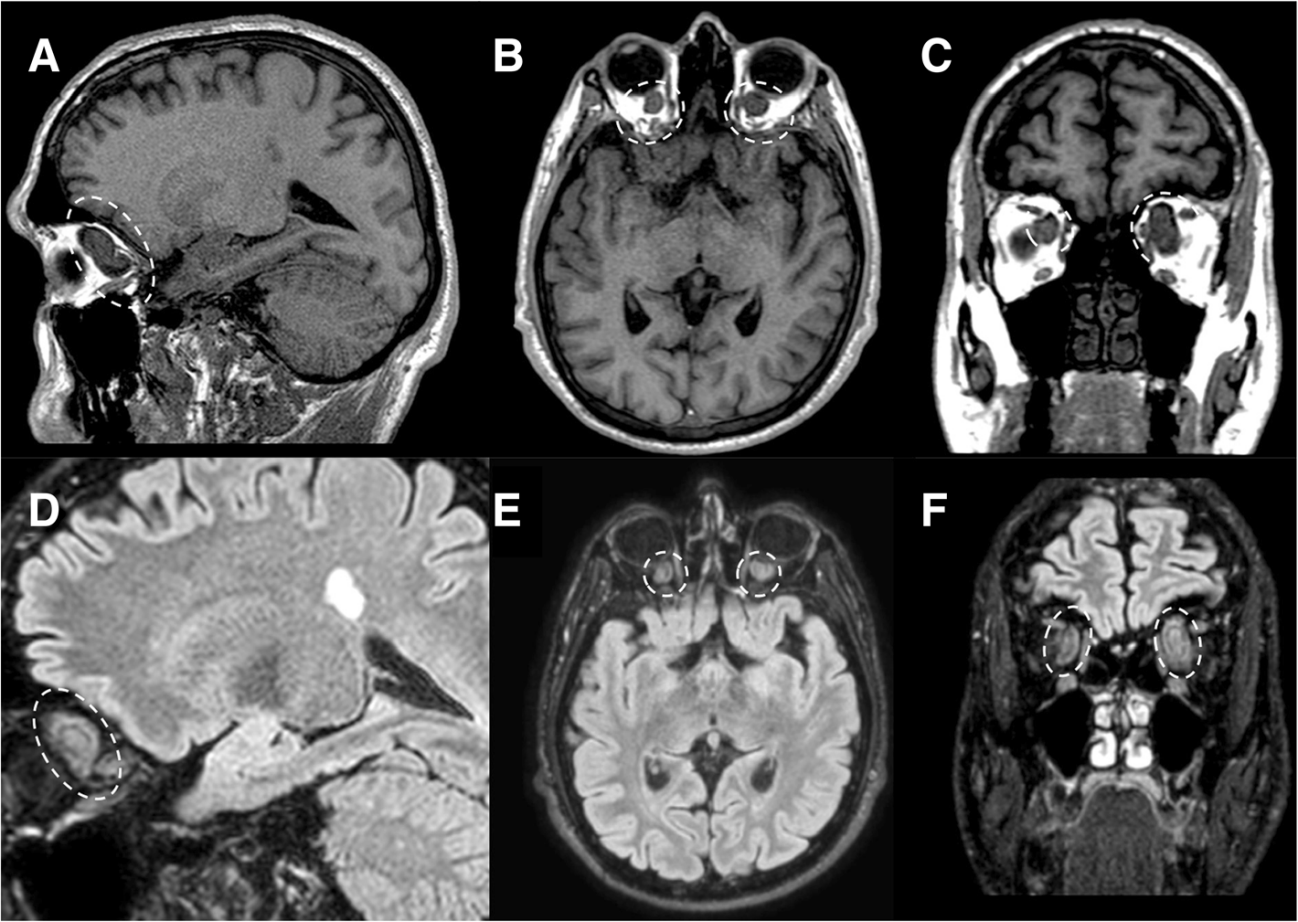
Optic nerve gliomas. MRI T1-weighted sagittal (a), axial (b), and coronal (c) images show bilateral intraorbital hypointense masses (dotted circles) centered on the optic nerve. FLAIR images (d–f) well depict the isointense masses causing enlargement of the optic nerves (dotted circles)
